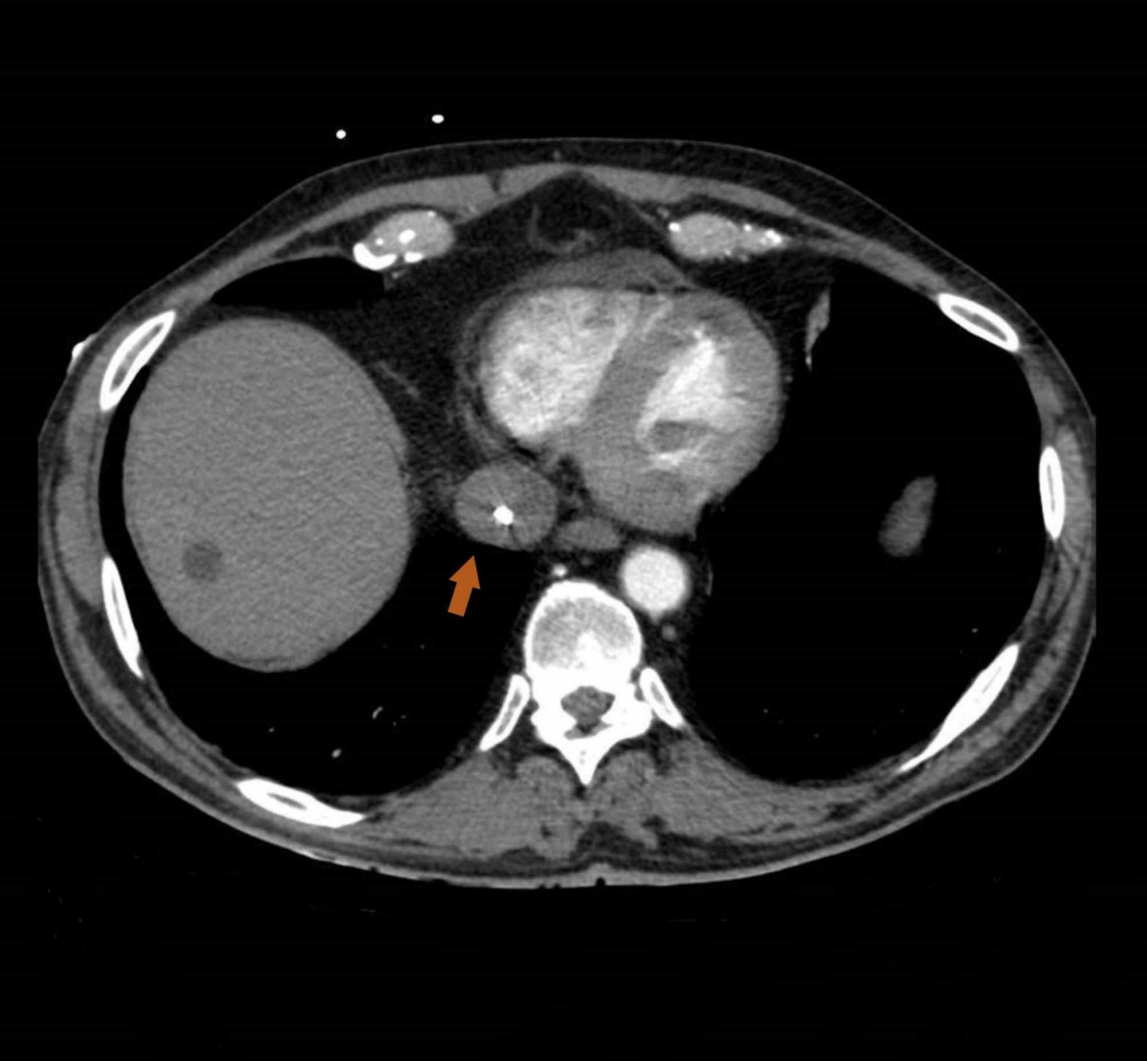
Catheter-related thrombus in IVC. CT scan showing a partial thrombus (arrow) surrounding the hemodialysis catheter in inferior vena cava (IVC).
